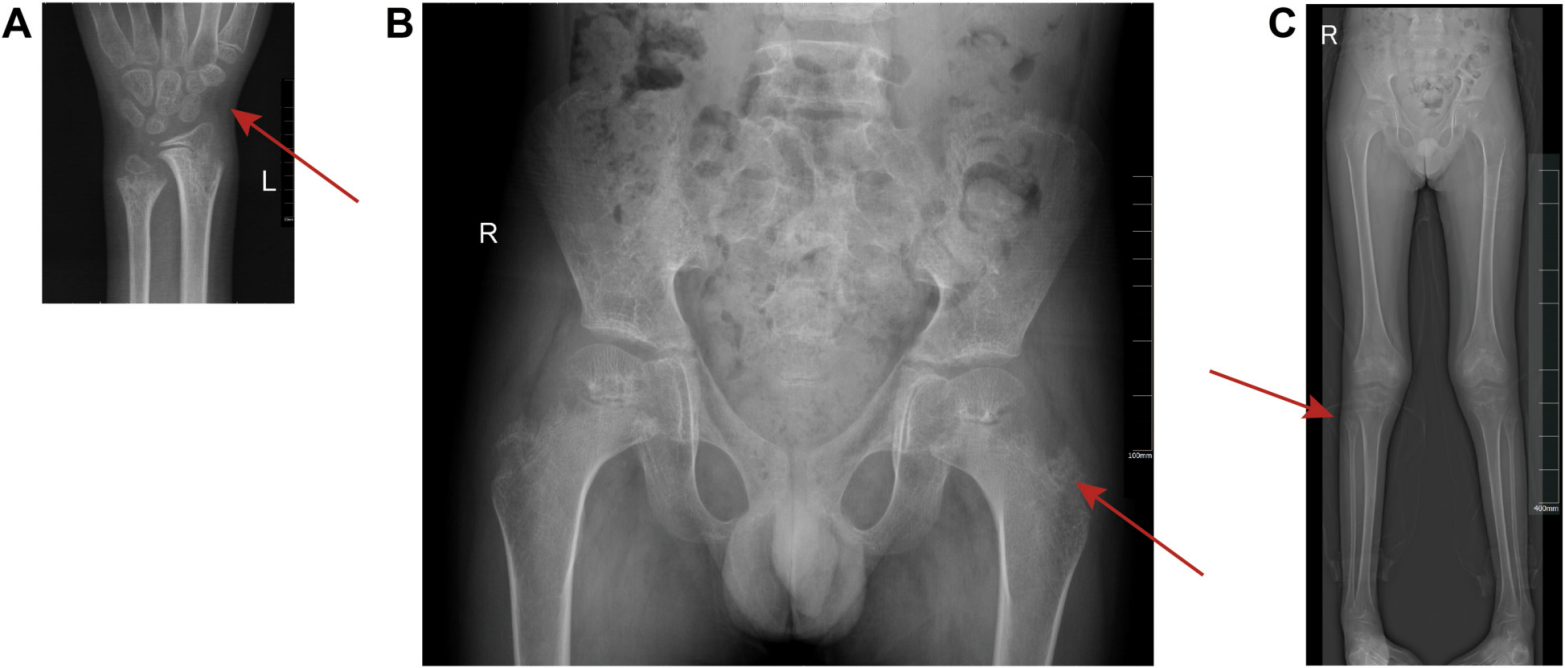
x-ray diagnostic images of children's skeletons. (A) Left Wrist x-ray: Anteroposterior view clearly shows the distal radius and ulna, highlighting metaphyseal irregularities and bone resorption. The red arrow indicates a cup-shaped deformity of the distal radius and delayed ossification of the carpal bones. (B) Pelvic x-ray: The frontal view captures the symmetry of the hips and pelvis, revealing developmental abnormalities such as asymmetric joint space and contour distortion. The red arrow marks acetabular roof tilting and reduced sacral bone density. (C) Lower Limb Full-Length x-ray: Anteroposterior projection displays the full extent of both femurs, tibiae, and fibulae, demonstrating generalized low bone density and metaphyseal changes. The red arrow highlights the bowing of the femur.
